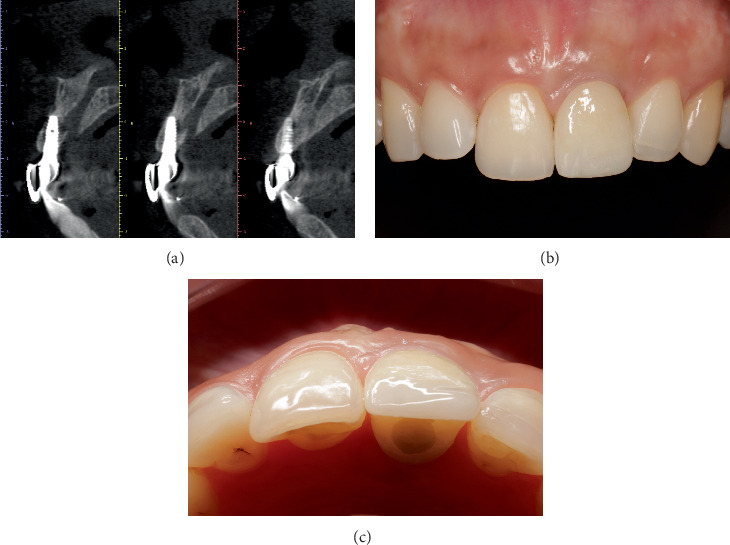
One-year follow-up. (a) CBCT control. (b, c) Frontal and occlusal views. Clinically, the recovery in volume and height of the peri-implant tissues associated with the prosthesis is observed.
